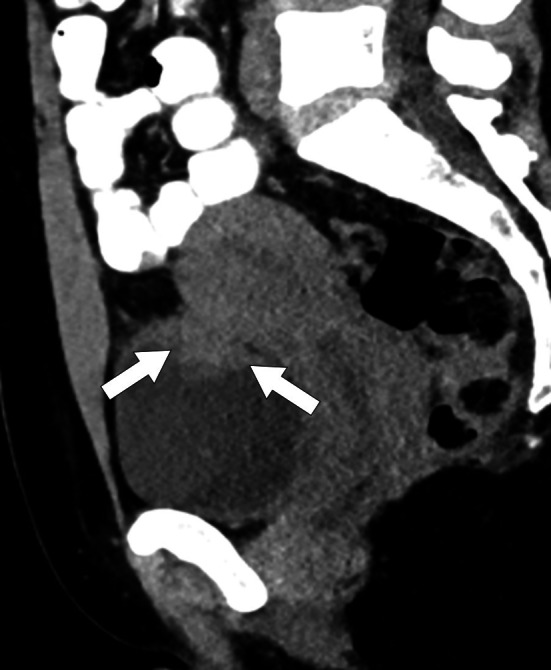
Deep endometriosis. A 40-year-old woman with no known medical history presenting with pelvic pain and hematuria. Sagittal precontrast CT image shows a nodular soft tissue mass located in the bladder dome inseparable from the anterior uterine wall. A neoplastic uterine mass invading the bladder was suspected. Surgical resection confirmed endometriosis
